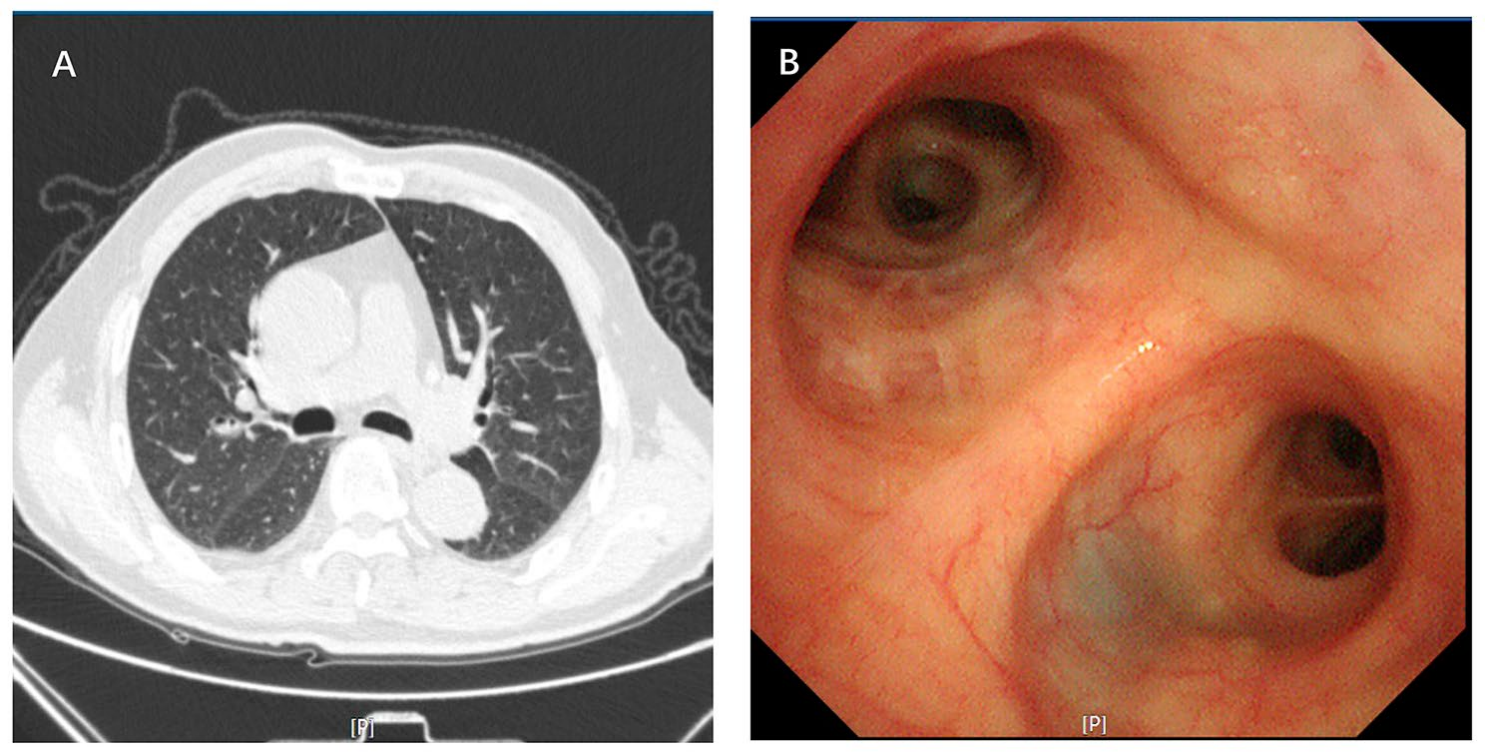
A: Repeat chest computed tomography scan on 20 February 2024; B: Tracheoscopy was reviewed on 21 February 2024
